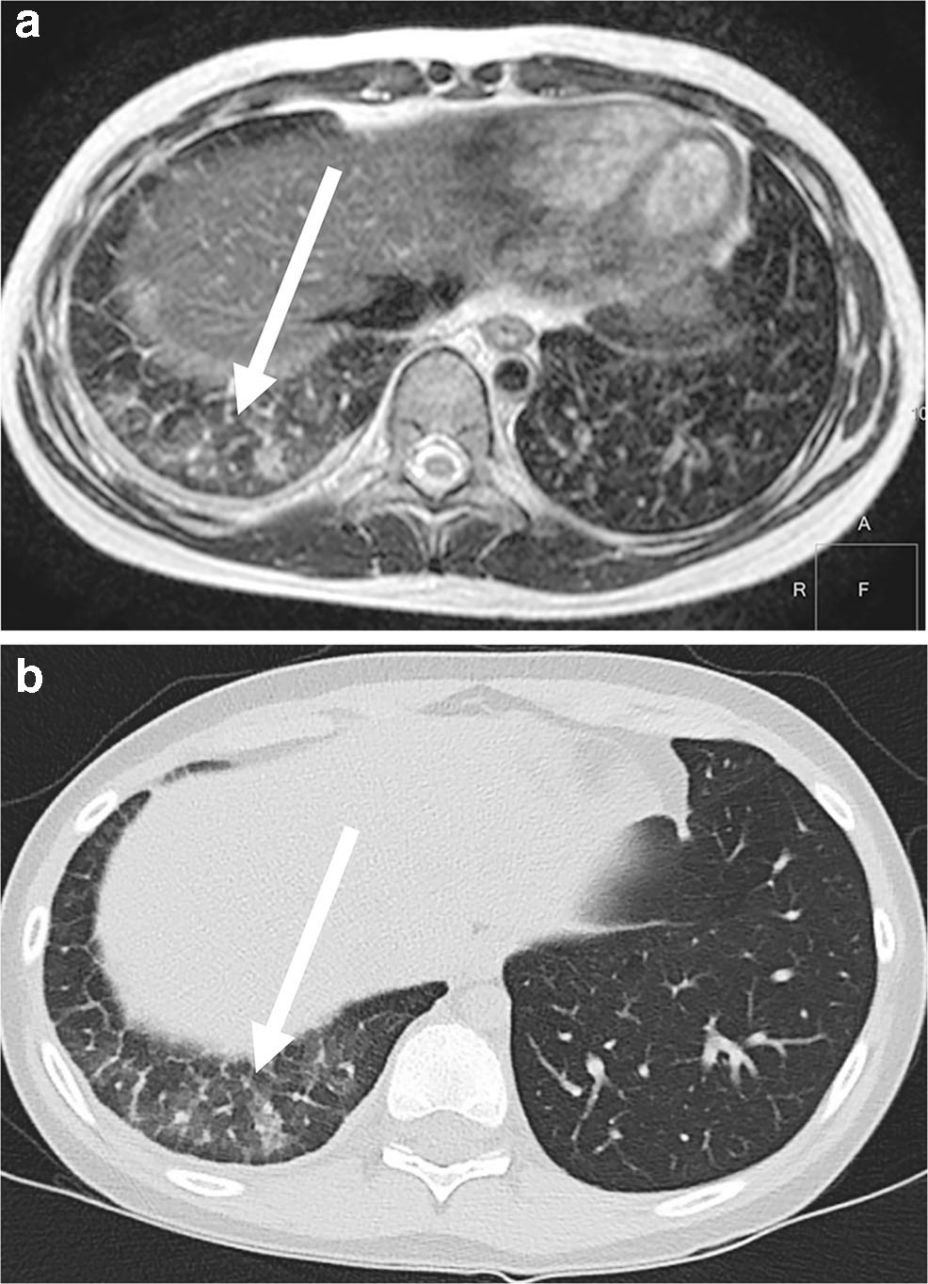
Mycoplasma infection in a 9-year-old boy. a Axial T2-weighted turbo spin-echo MR image (parameters in Table 2) shows local interstitial fluid collection from mycoplasma infection and interstitial reaction (*arrow*) in the right dorsal recess. b Axial CT shows corresponding findings (*arrow*)
